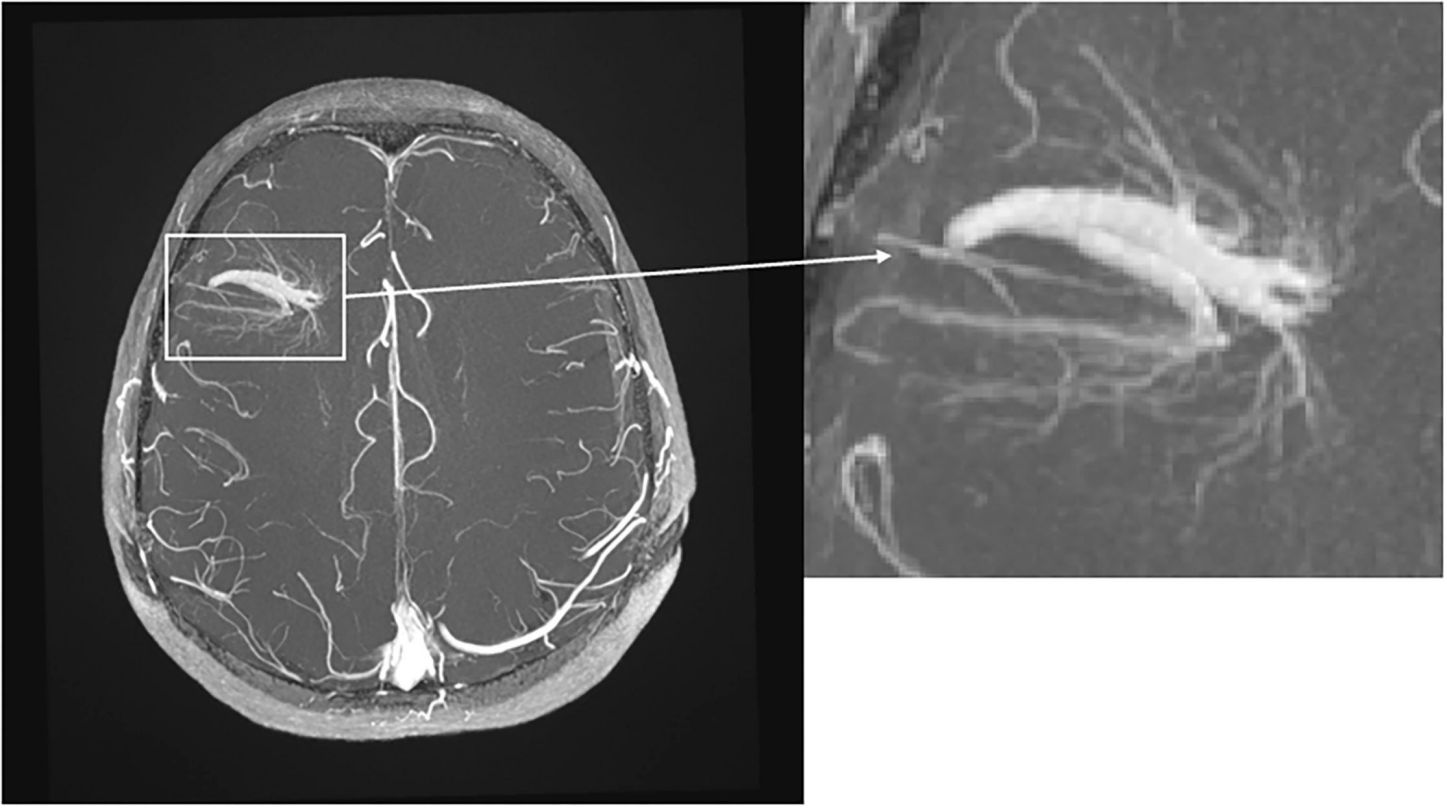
Transverse T1-weighted TOF MRI with intravenous contrast. Note the big DVA with two collecting veins in the right frontal lobe with “umbrella sign.”
